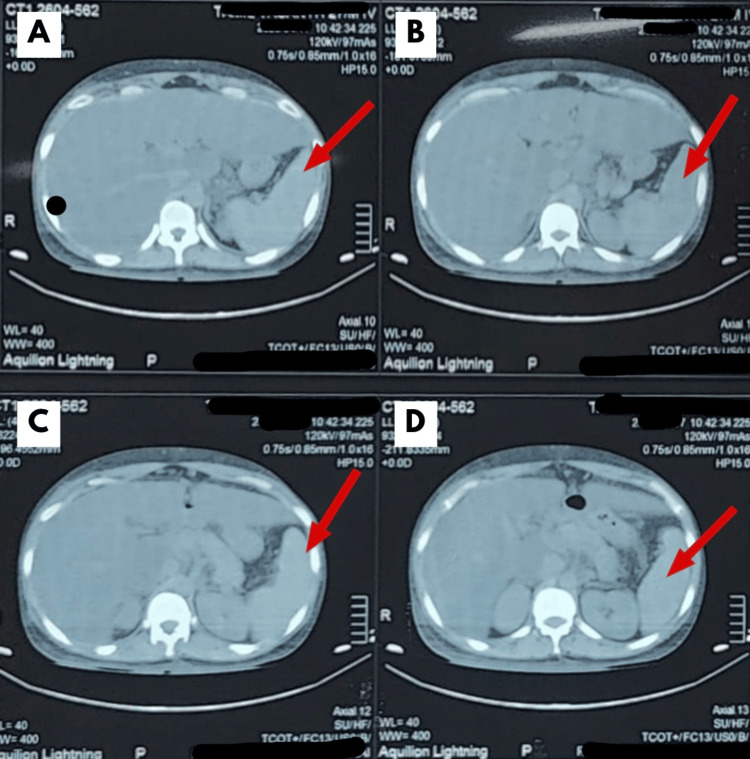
Axial contrast-enhanced CT scan of the abdomen showing an enlarged spleen (indicated by the red arrow) (A-D) Axial CT images at different levels demonstrate spleenomegaly, as indicated by red arrows. Splenomegaly in autoimmune hemolytic anemia occurs due to increased sequestration and extravascular destruction of antibody-coated red blood cells within the spleen, reflecting ongoing hemolysis and increased splenic activity CT: computed tomography
